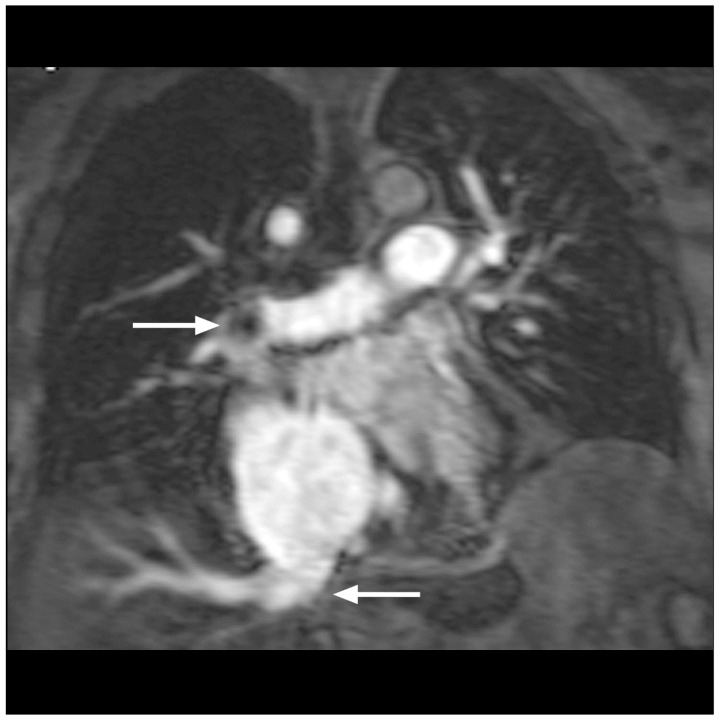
Pulmonary MRA in a 76-year-old male patient. After an initial CT examination and thrombolysis, the MRA control examination showed a residual central thrombus in the right pulmonary artery (upper arrow) with perfusion deficit. Right heart insufficiency with reflux of contrast medium in the inferior vena cava (lower arrow). Moderate examination quality.
